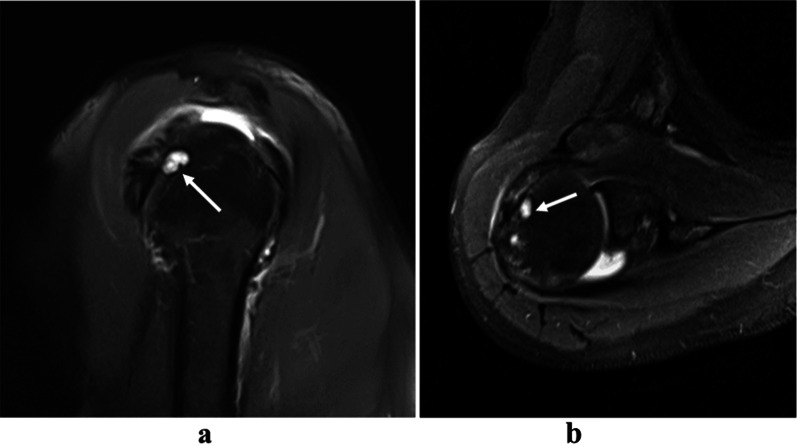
Lesser tuberosity cysts (LTC) on oblique sagittal (a) and axial (b) IM-WI MRI show well-defined fluid-like cystic lesion within the lesser tuberosity (white arrow)
